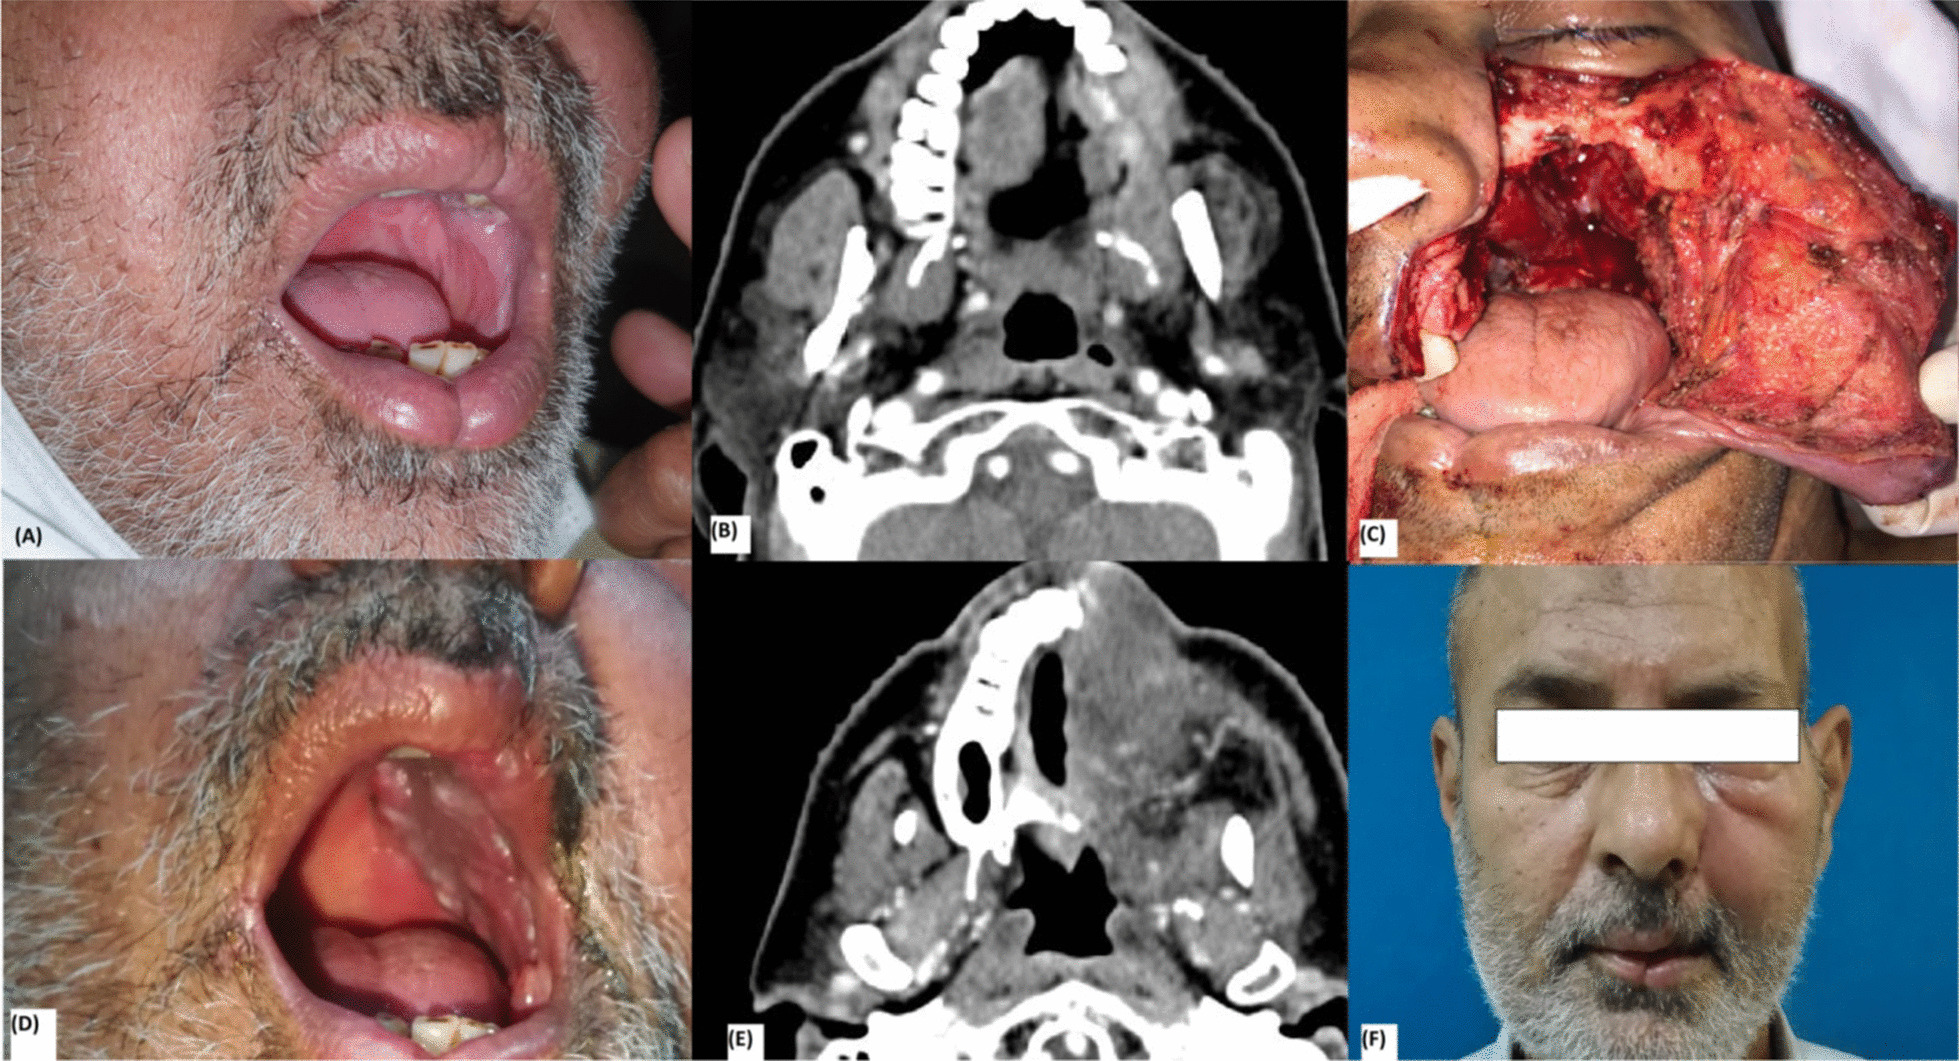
Case 4 a Middle aged male with biopsy proven well differentiated squamous cell carcinoma of the left maxilla. b CT scan with contrast showing enhancement in the left maxilla sinus, left sided palate with destruction of the left maxillary arch. c Picture showing a moderate volume with large surface area defect after wide local excision of the tumor (subtotal maxillectomy) including resection of maxillary arch, palate and anterior and lateral walls with preservation of the orbital floor. d Four weeks follow up picture showing good mucosalisation of the temporal muscle flap. e Follow up CT scan of the patient showing temporalis muscle flap filling the defect. f Late follow up picture, patient has good facial contours with mild temporal hollowing
